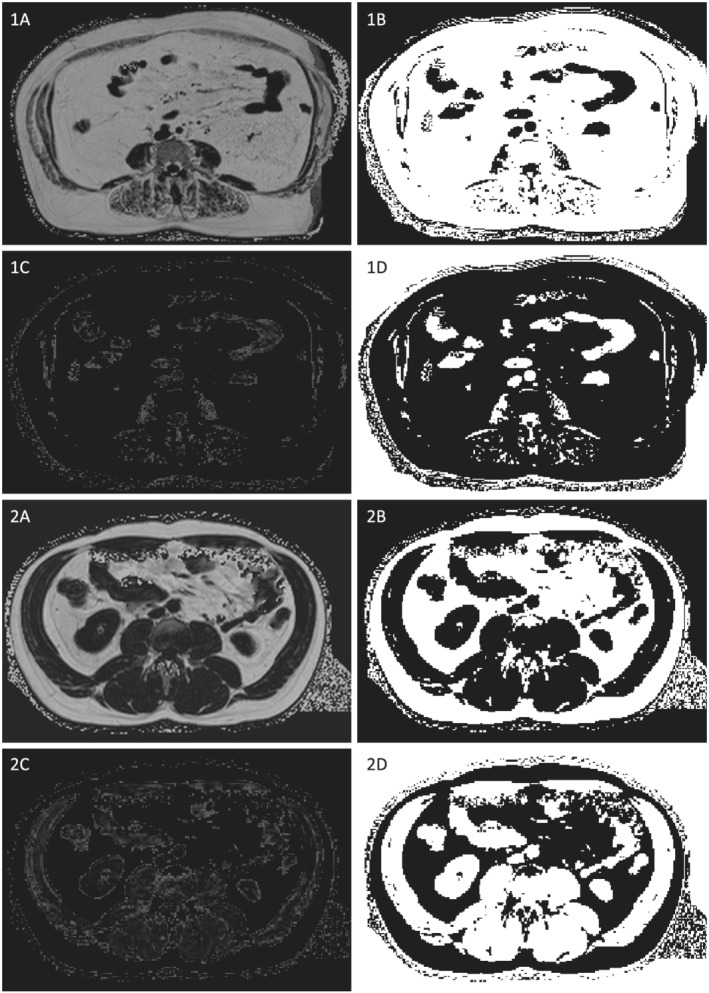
Example of an obese (A) and normal weight (B) subject with lower (A) and higher (B) total and fat‐free abdominal skeletal muscle mass as cross‐sectional areas by MRI (post‐processed with Matlab_R2017a). (1) Original proton‐density fat‐fraction map. (2) Segmentation of adipose tissue. (3) Segmentation of intra (myo)cellular lipids. (4) Segmentation of fat‐free muscle mass.
